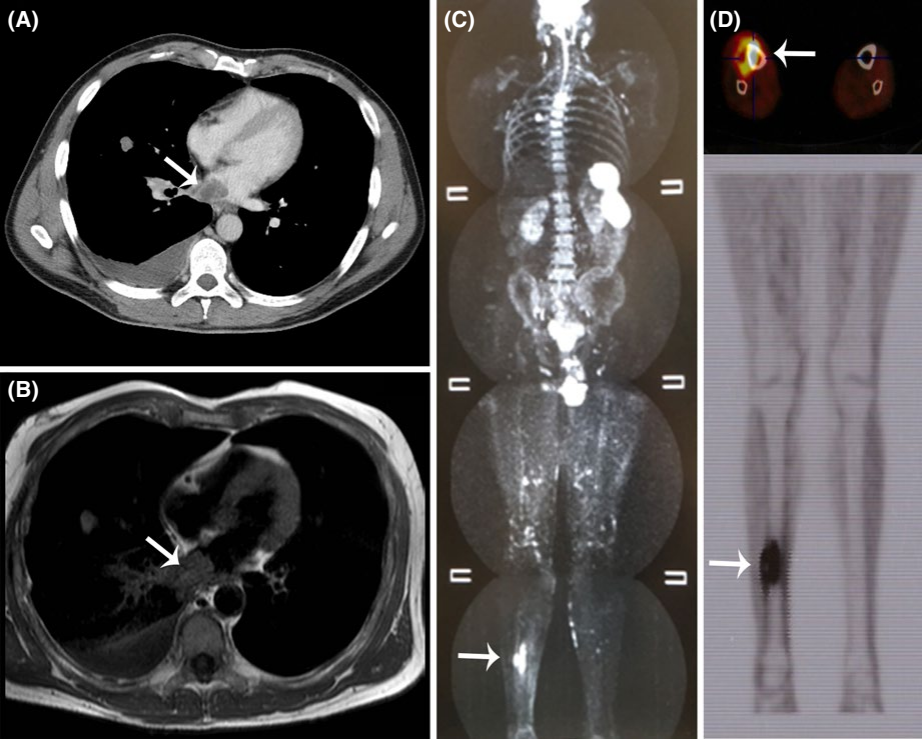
CT (Panel A) and MRI scan (Panel B) showing a metastasis in the left cardiac atrium; whole body MRI (Panel C) and axial/coronal PET scan (Panel D) detecting a metastasis in the right tibia
